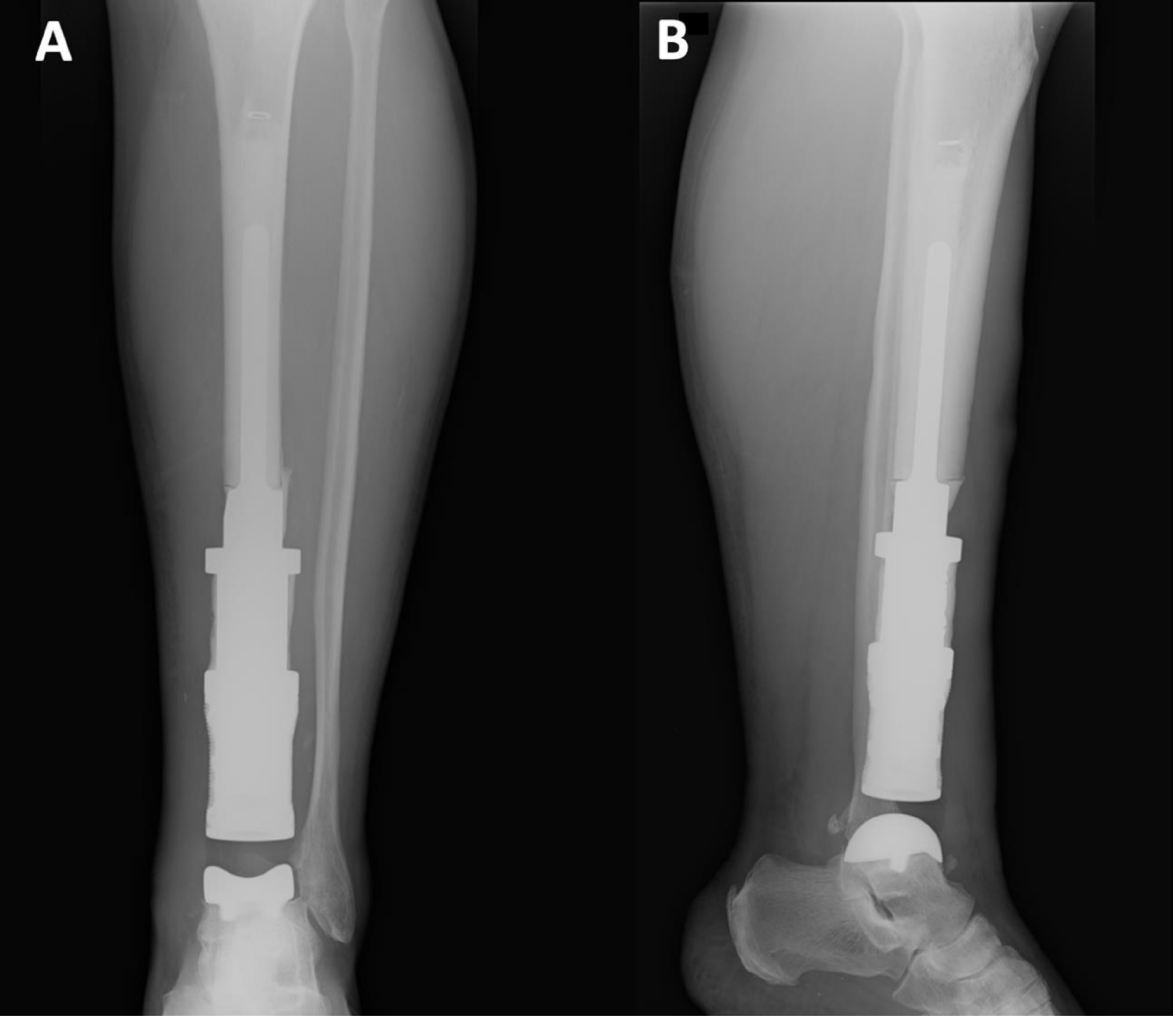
X-rays of the Case 1’s distal tibia and ankle megaprosthesis in an (A) anteroposterior and (B) lateral view.
